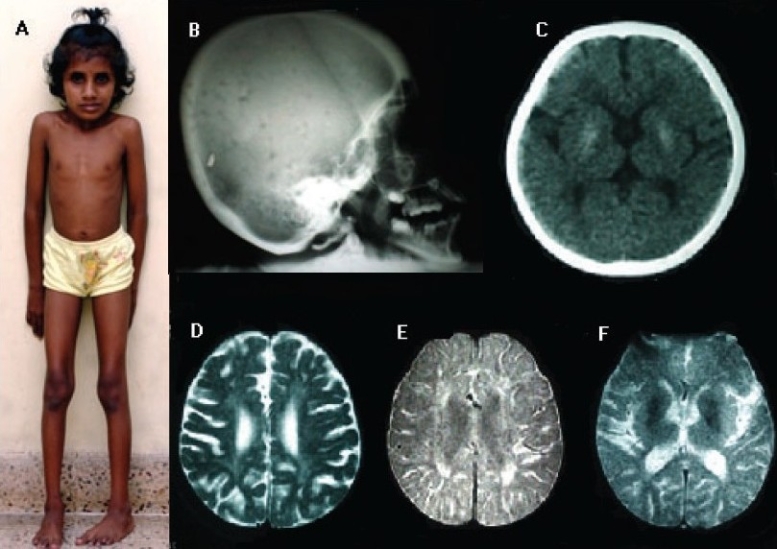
A: Emaciated, dwarf child with progeric face (‘cachectic dwarfism’). Also note the foot dystonia; B: Lateral skull x-ray showing calvarial thickening; C: Axial CT scan (at 5 years of age) showing bilateral basal ganglia calcifications; D, E, and F: Axial MRI scans of the brain (T2WI, proton density image, and gradient spin-echo image, respectively) showing generalized atrophy, symmetrical white matter hyperintensities, and basal ganglia calcification
